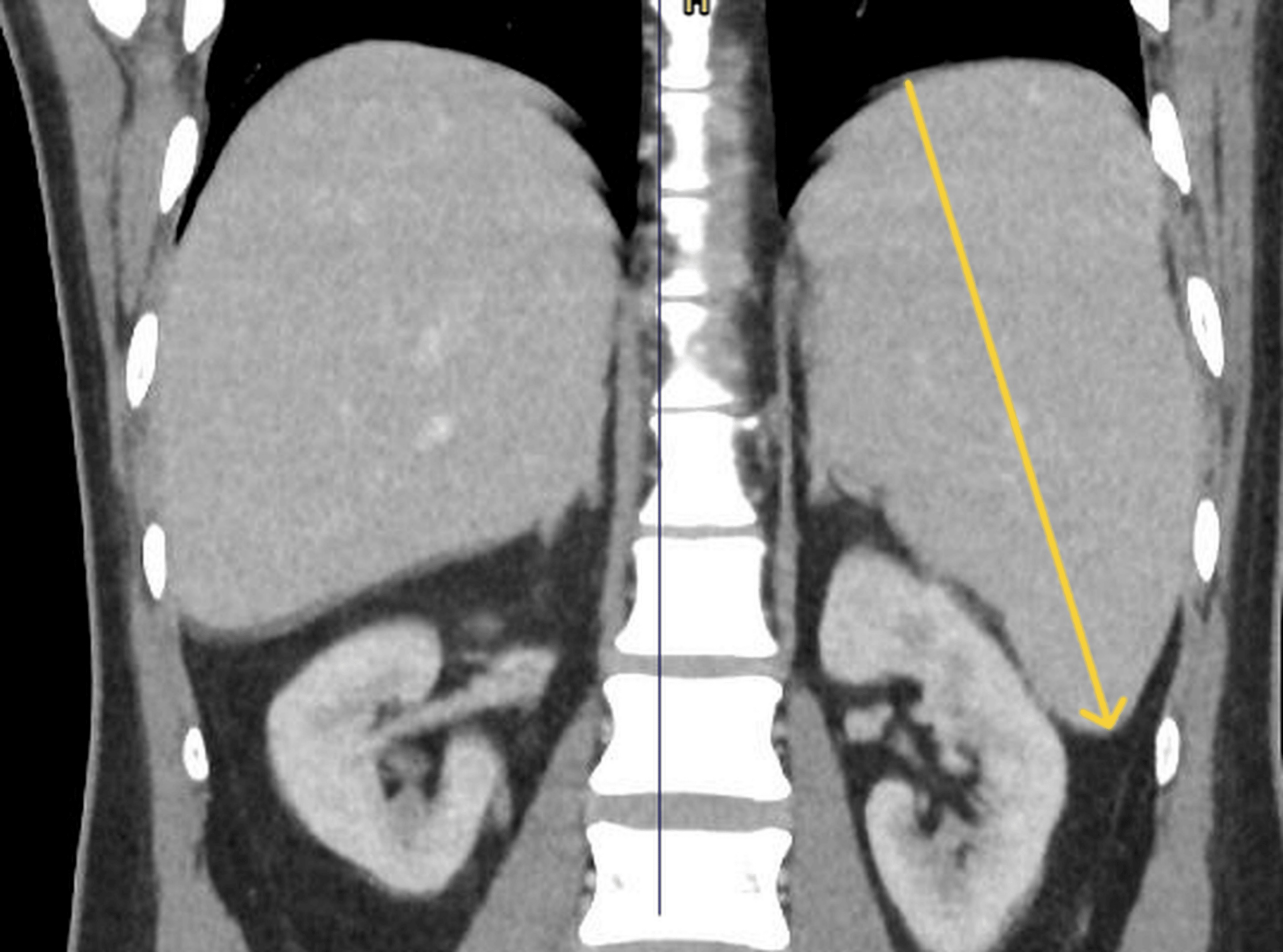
Computed tomography of the abdomen (coronal view) showing splenomegaly. Yellow arrow showing the splenomegaly.
